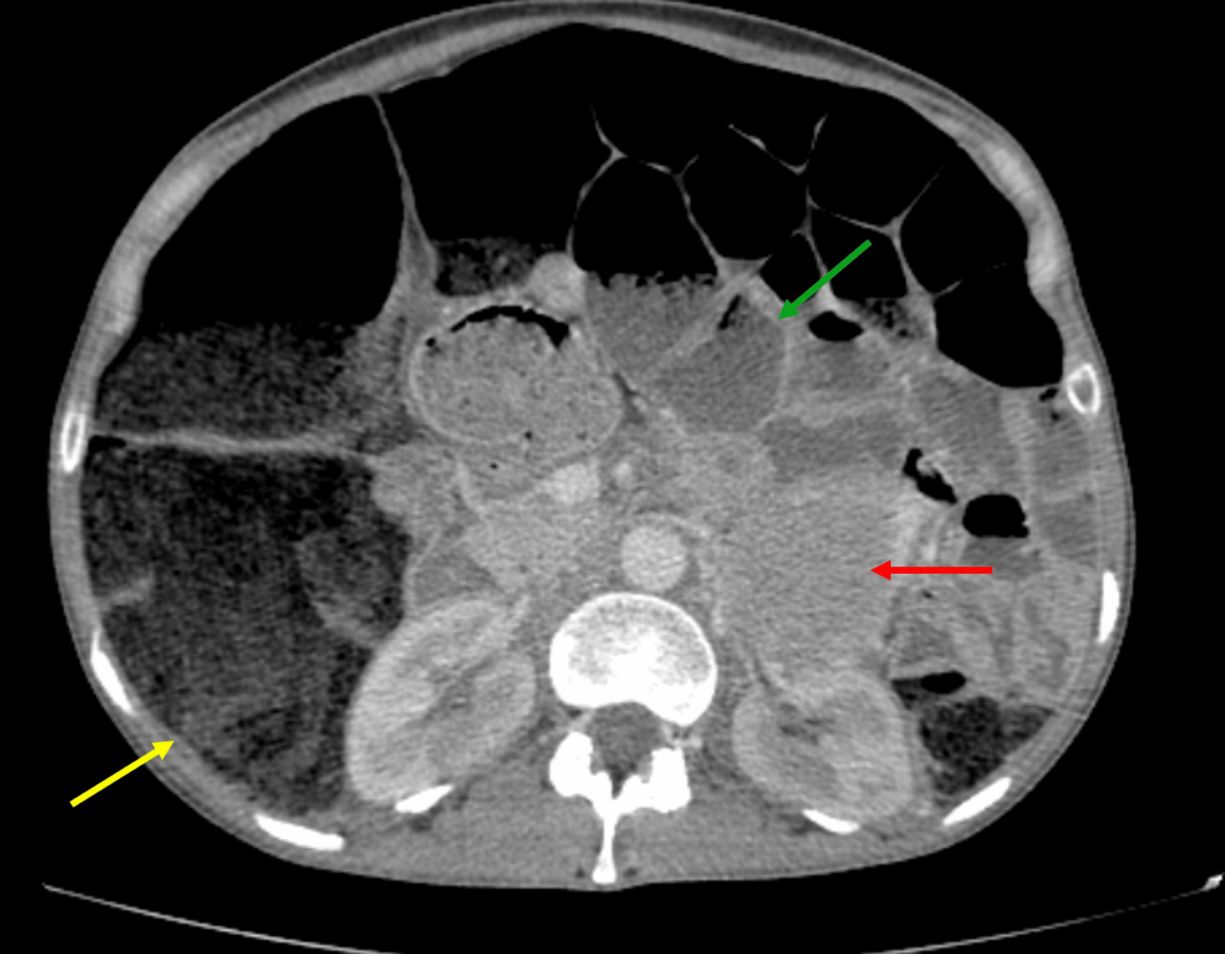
Contrast-enhanced axial abdominal CT showing marked colonic (yellow arrow) and small-bowel distension (green arrow), with a heterogeneous left adrenal mass (red arrow)
